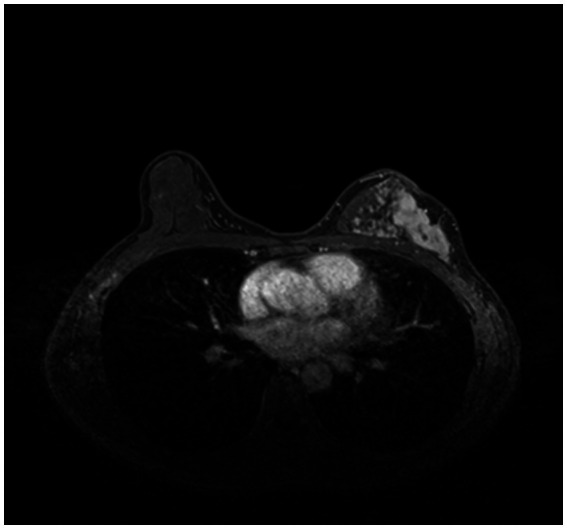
An example of the 361 labeled DCE-MRI samples in our dataset.
